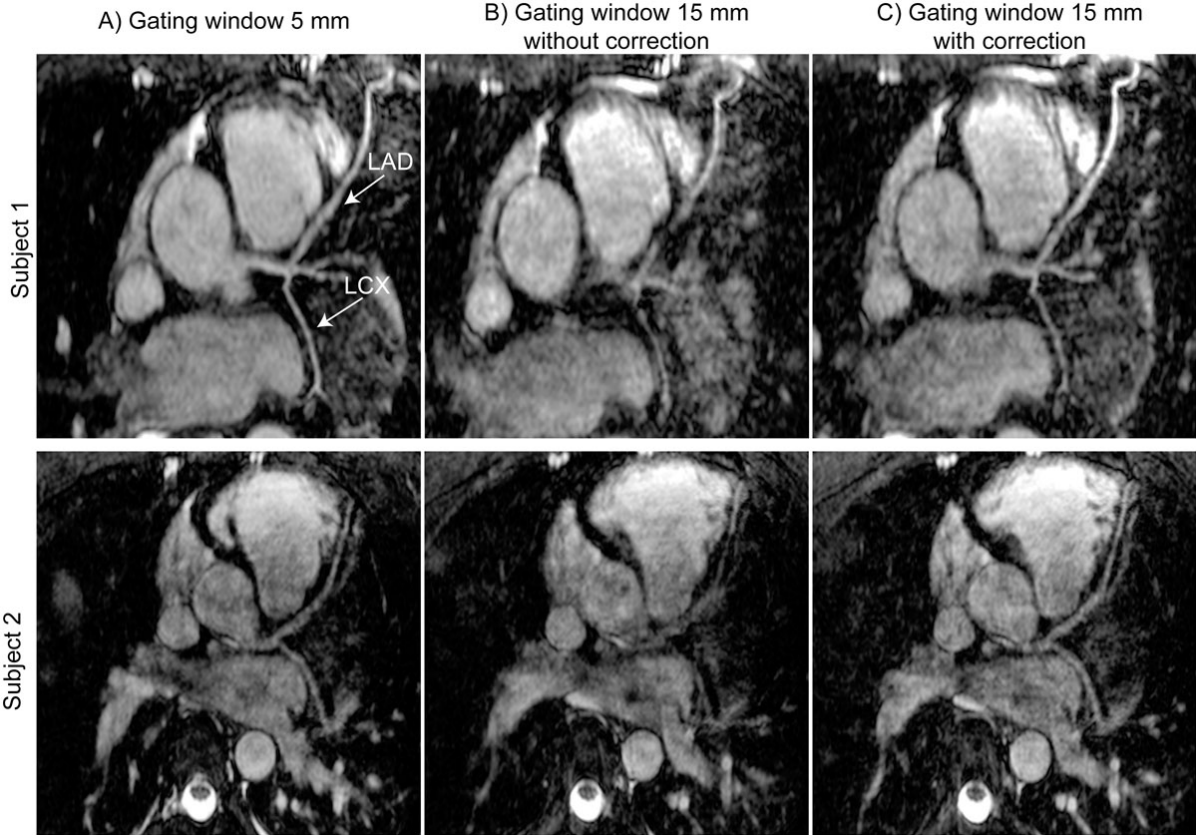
Reformatted whole-heart coronary MRI acquired from three healthy subjects using A) a right hemi-diaphragm (RHD) navigator with a 5 mm gating window; B) a RHD navigator with 15 mm gating window without correction and C) a RHD navigator with 15 mm gating window with correction. Arrows identify the left anterior descending (LAD) and left circumflex (LCX) arteries.
